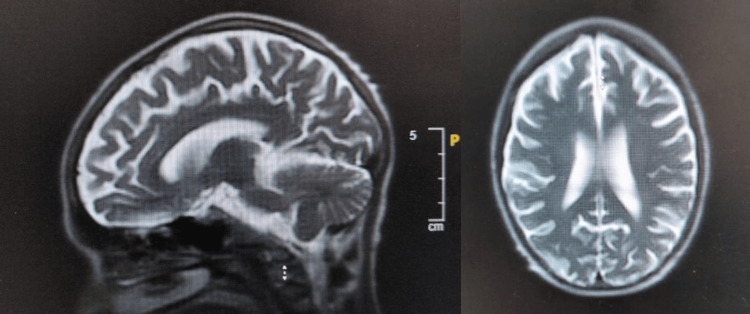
MRI Brain (T2-weighted sequences) Left: Sagittal view showing diffuse cerebral and cerebellar volume loss disproportionate to age. Right: Axial view demonstrating global sulcal widening and mild ventricular prominence without a focal lesion.
